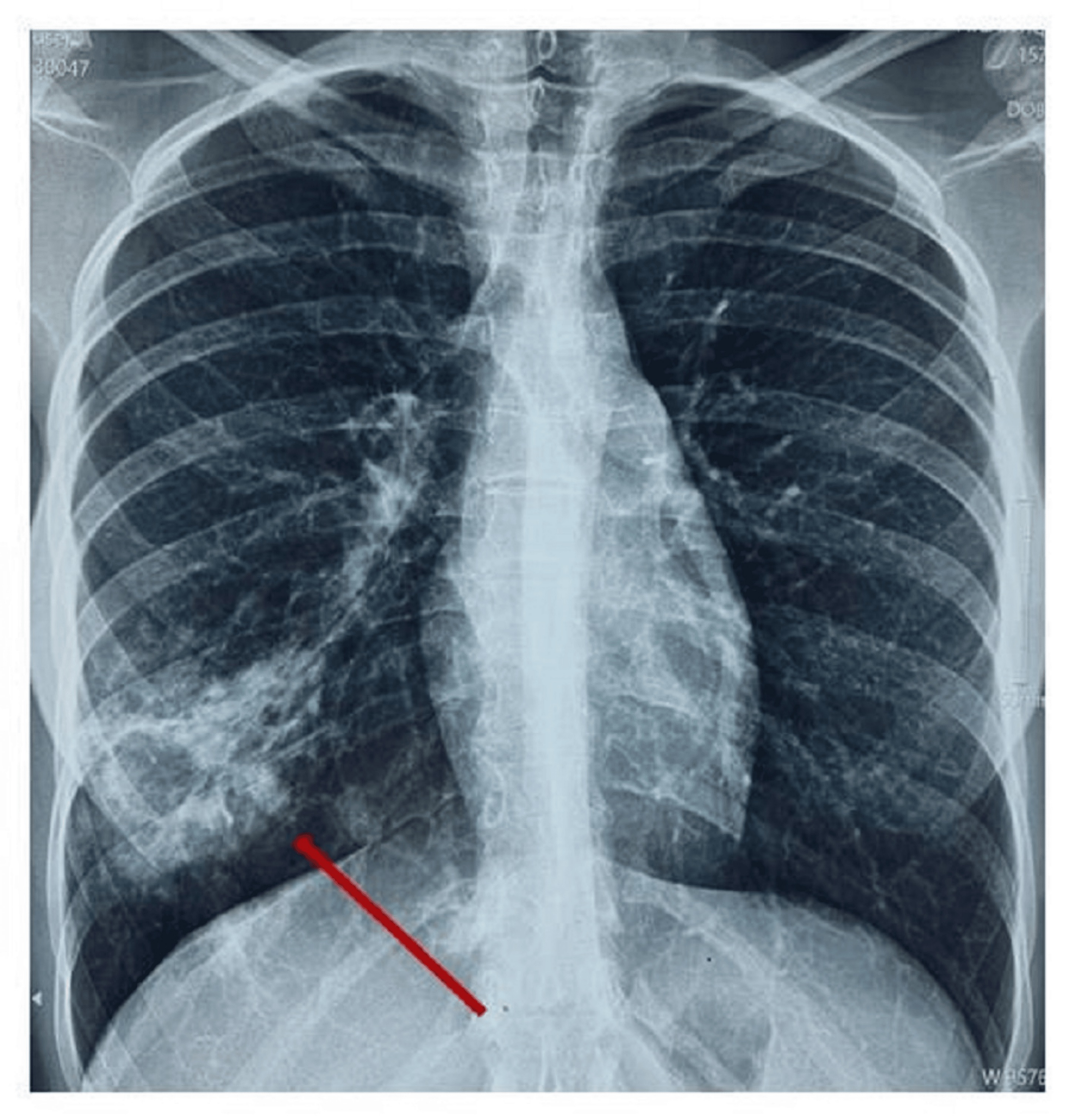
Chest X-ray image showing a cavitary lesion in the right lower lobe (red arrow).
